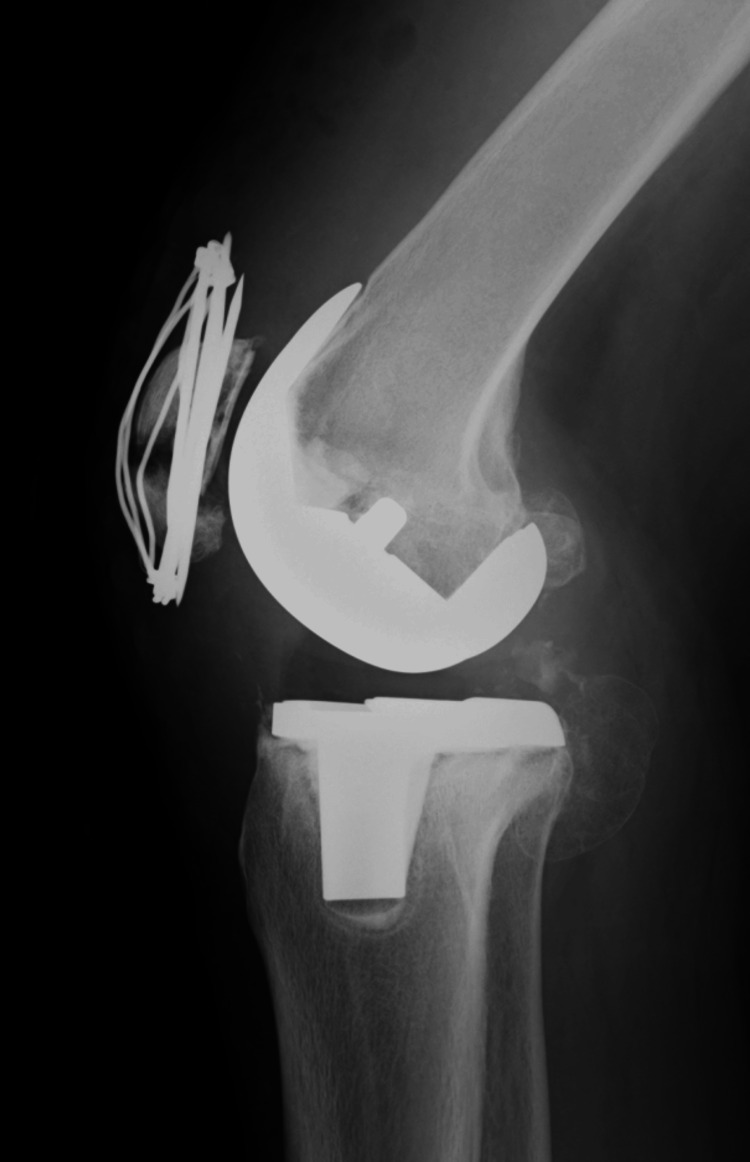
Postoperative X-ray following refracture surgery. At this time, revision osteosynthesis was performed using the AI wiring system (Aimedic MMT, Tokyo, Japan). Again, no loosening of the patellar component was observed during the operation and postoperative X-ray.
